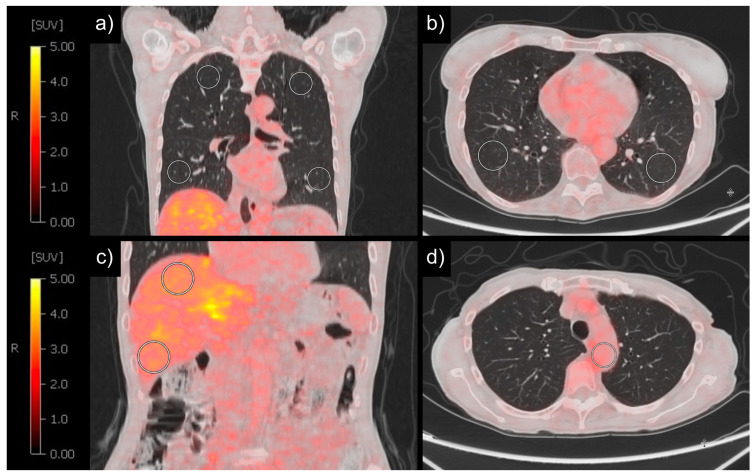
Example of PET/CT analysis with spheres. SUV measurement with spheres with 30 mm diameter in lung in coronal view (a), lung in axial view (b), and liver in coronal view (c). SUV measurement with one sphere and diameter depending on aorta size in blood localized in aorta in axial view (d).
